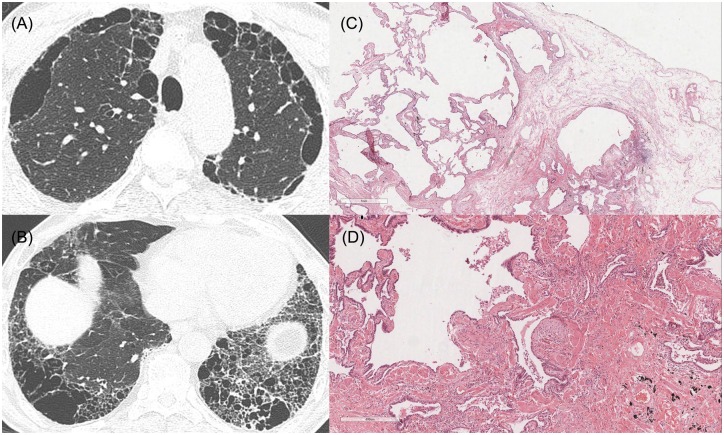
HRCT images and pathologic features of a 67-year-old man who was diagnosed with SRIF in pathology. (A), (B) HRCT shows upper lobe-predominant paraseptal emphysema, and asymmetric, inhomogeneous honeycombing with bullae in the lower lobes. (C, D) Pathologic features show emphysematous change, interstitial fibrosis and dense fibrosis with architectural distortion and fibroblastic focus (x20, x40). He was diagnosed with SRIF by multidisciplinary discussion, because also he was not suspicious to have IPF in clinical information. The pSRIF score was 2.
